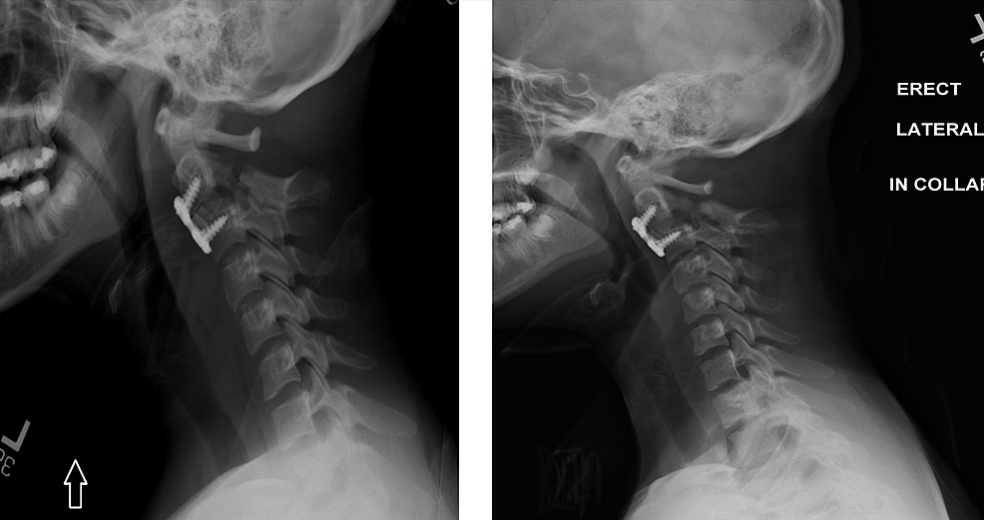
Post-operative X-ray (lateral view) 8 weeks following C2 to C3 ACDF. Stable alignment of the vertebral body and hardware is present, but posterior pars defect has not shown any evidence of bony healing (left). Follow-up imaging shows bony healing and stable hardware alignment (right).
